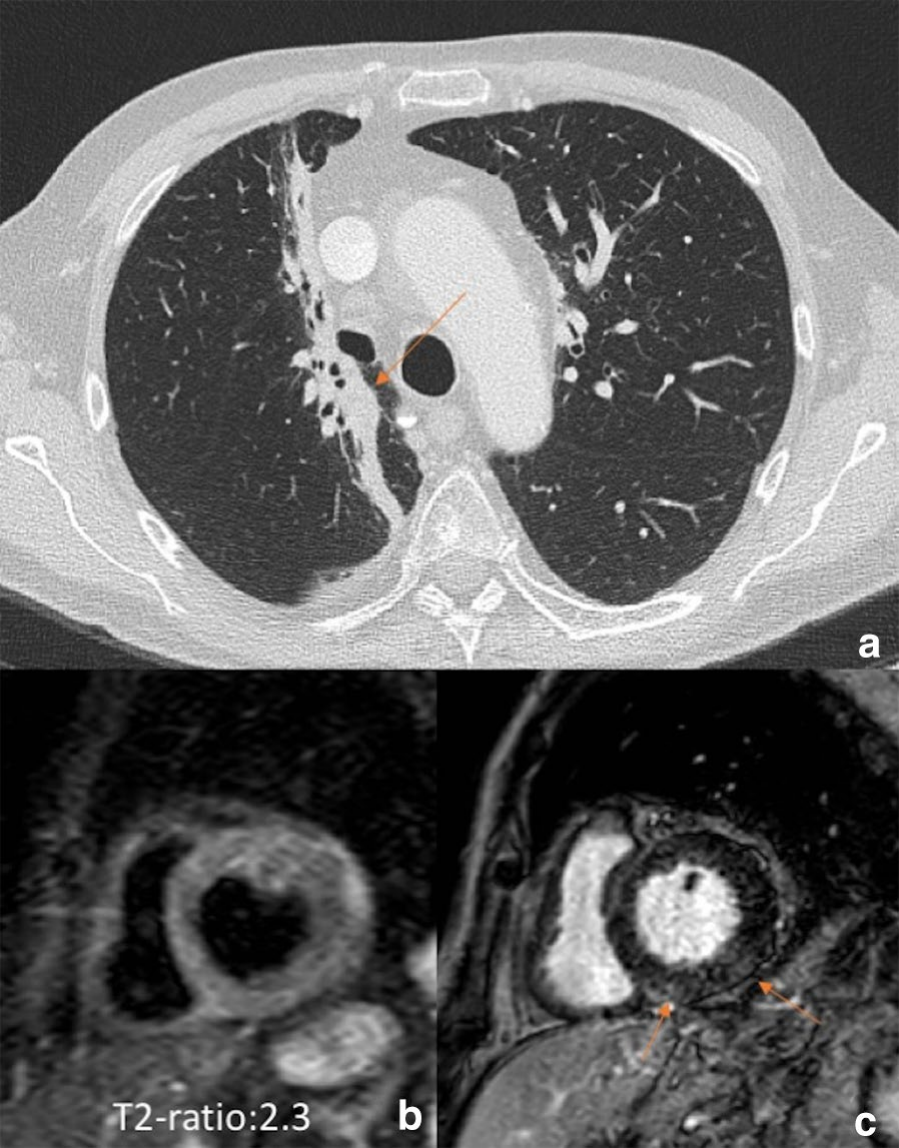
Myocarditis associated to immune checkpoint inhibitor in a patient with lung cancer. A 67-year-old man with advanced lung adenocarcinoma (stage IIIB) was initially treated with ChT/RT. Axial CT image (a) showing right lung RT-related changes around the primitive cancer (arrow). Due to an incomplete response, treatment with immune checkpoint inhibitor was administered. The patient attended was admitted four months later at the emergency department for acute dyspnea associated to increased high sensitive troponin T (113 ng/L; normal value < 14 ng/L). Cardiac MR showed a left ventricle with normal volume (83 mL/m^2^) but with a slight depression of the ejection fraction (47%) associated to a diffuse hyperintensity on STIR image and increased T2-ratio (b) suggestive for diffuse edema and a shadowed late gadolinium enhancement in the inferior wall with non-ischemic pattern. MR findings indicated active myocarditis which was confirmed at endomyocardial biopsy
